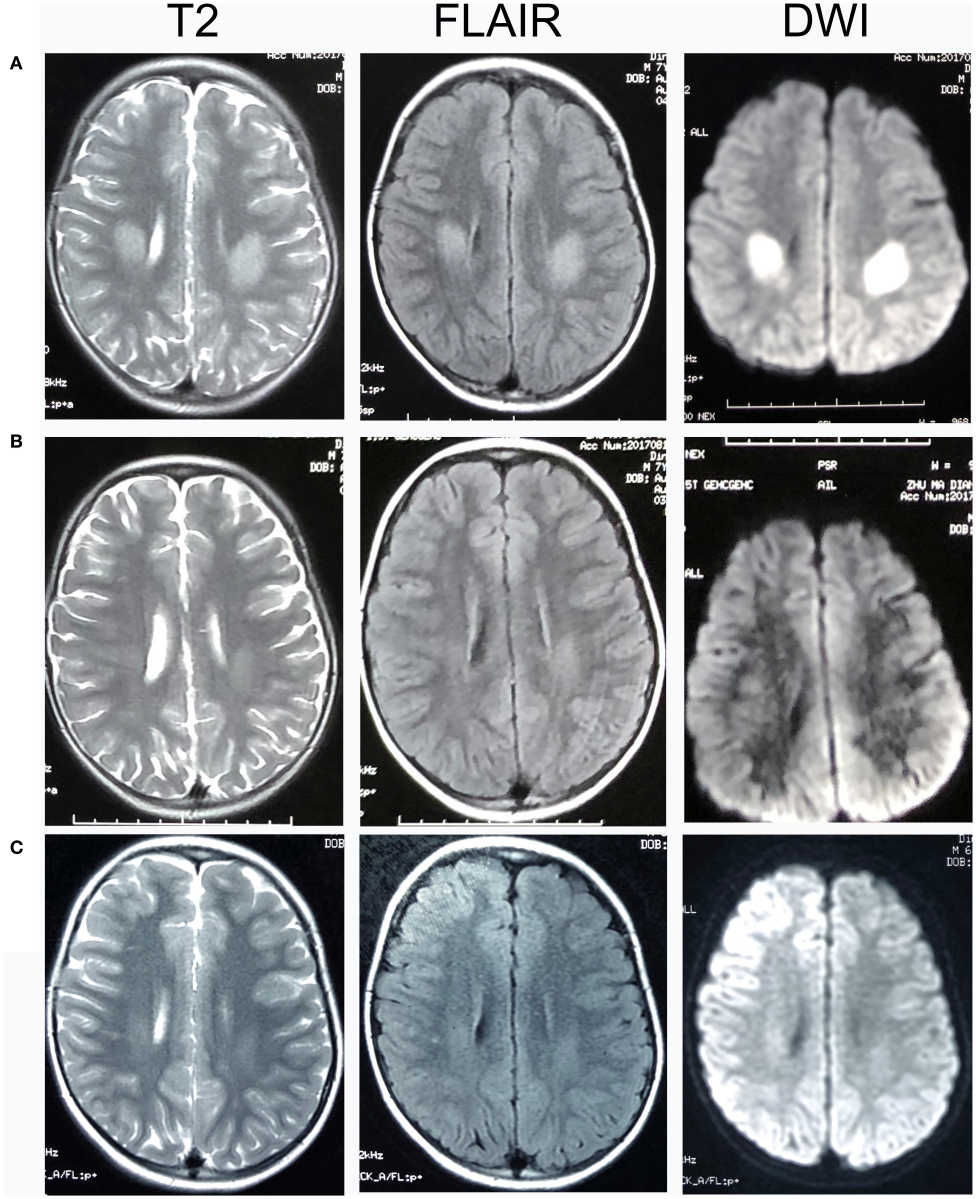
Head MRI of Patient 11. T2-, fluid-attenuated inversion recovery (FLAIR), and diffusion-weighted magnetic resonance imaging (DWI) images of Patient 11. (A) 2 days after the onset of his neurological syndrome; (B) 8 days after onset; (C) 1 month after onset. There were bilateral centrum semiovale T2 and DWI hyperintensities 2 days after onset, which significantly decreased on day 8 and at 1 month.
